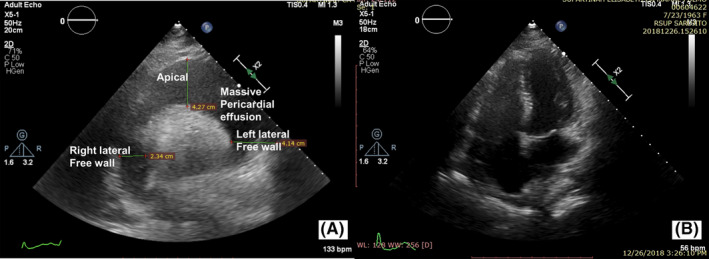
Echocardiography. A, September 2018: massive pericardial effusion during cardiac tamponade episode with ejection fraction of 50% and a decreased right ventricle function. B, December 2018: disappearance of pericardial effusion after several cycles of weekly docetaxel treatment
